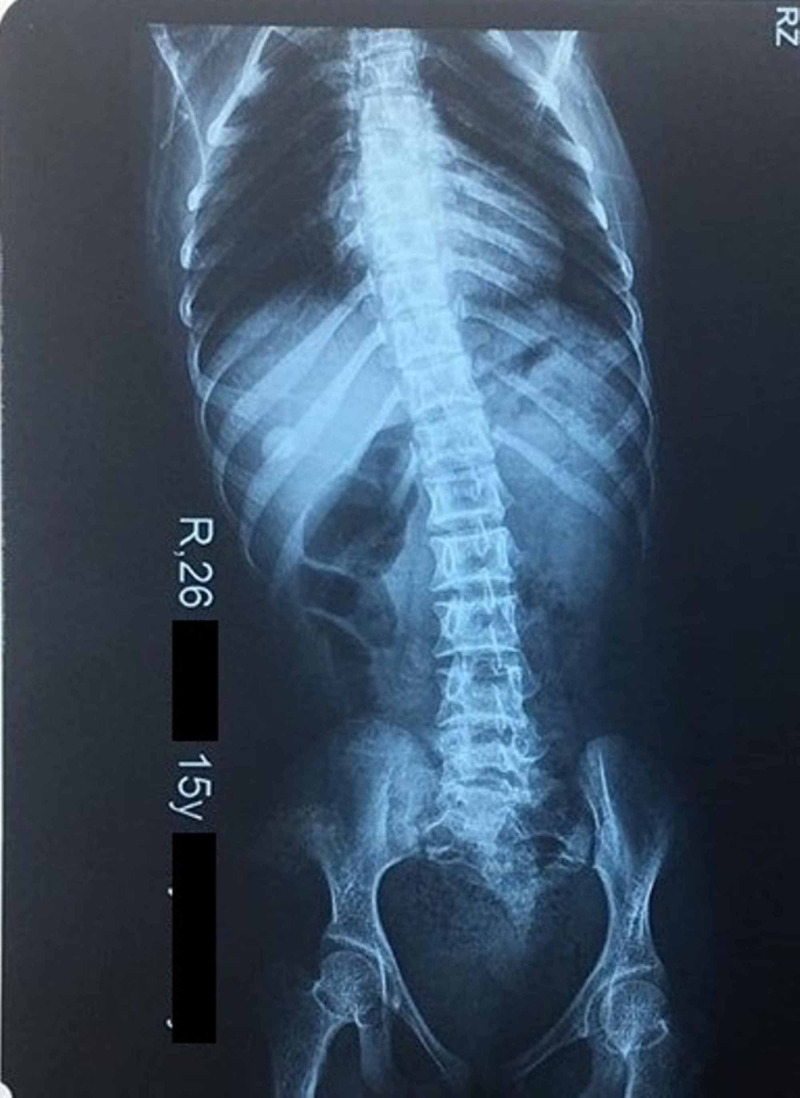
Case one: thoracic scoliosis as evident on the scoliosis X-ray
